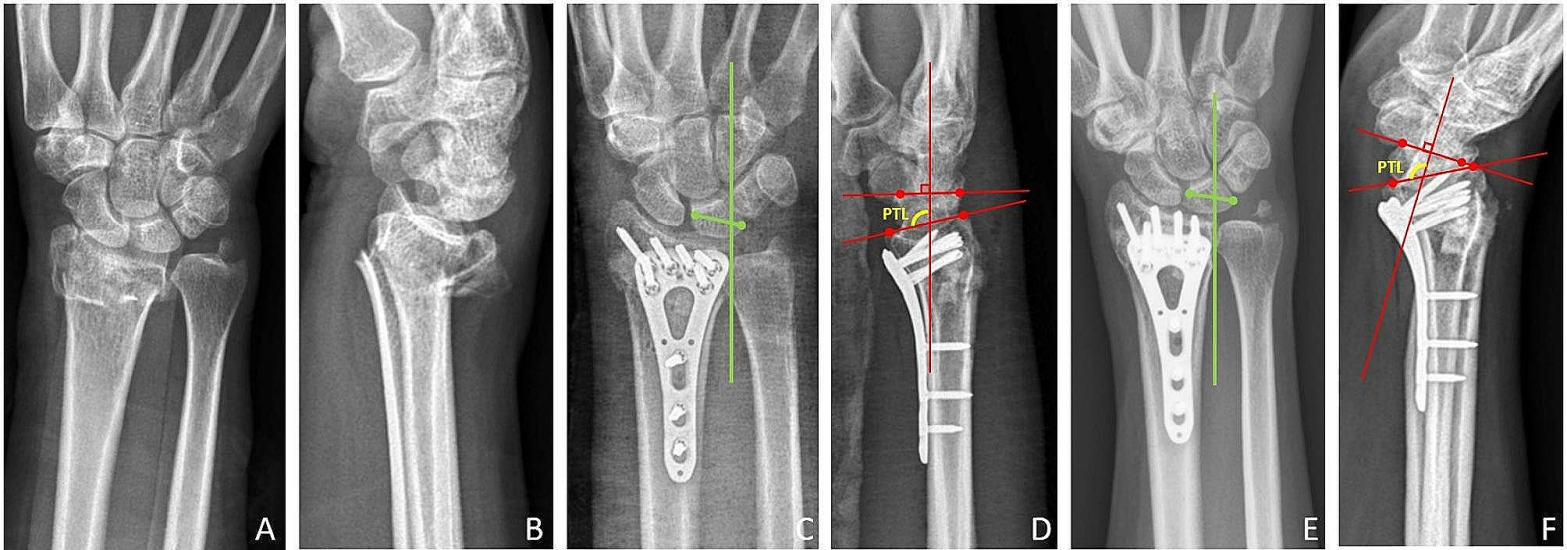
A 63-year-old woman with right distal radius fracture. Radiographs showing (A) and (B) the fracture before treatment; (C) and (D) after open reduction and internal fixation. (E) and (F) Radiographs taken 3 months postoperatively, revealing ulnar carpal translation (UCT) and rotatory palmar subluxation of the lunate (RPSL) as evidenced by an increase in the palmar tilt-lunate (PTL) angle
